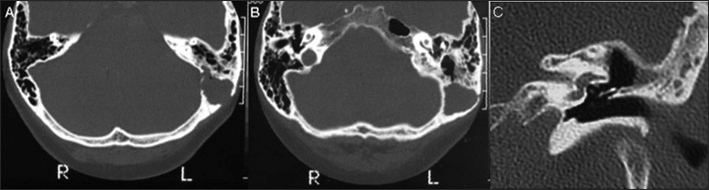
A-B: CT of the temporal bones. Axial images showed a hypoattenuating and expansive lesion in the left mastoid process eroding its medial and lateral walls, adjacent to the left sigmoid sinus. The remaining structures were unremarkable. C: Follow-up coronal CT image of the left temporal bone did not reveal recurrence and showed a large surgical cavity in the mastoid process communicating antrum with tympanic cavity.
